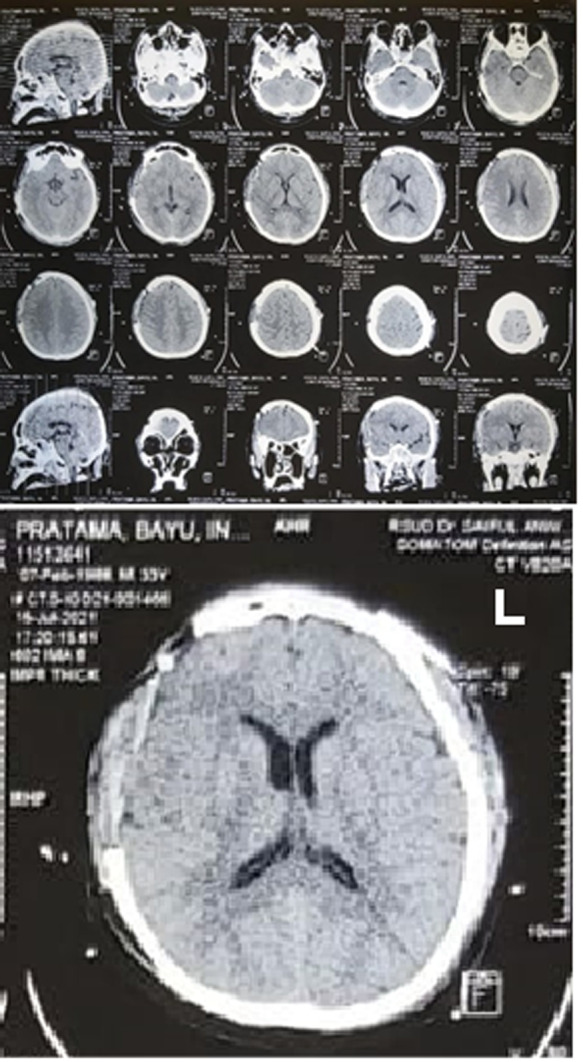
head CT after surgery showing improvement where the hematoma has been evacuated
